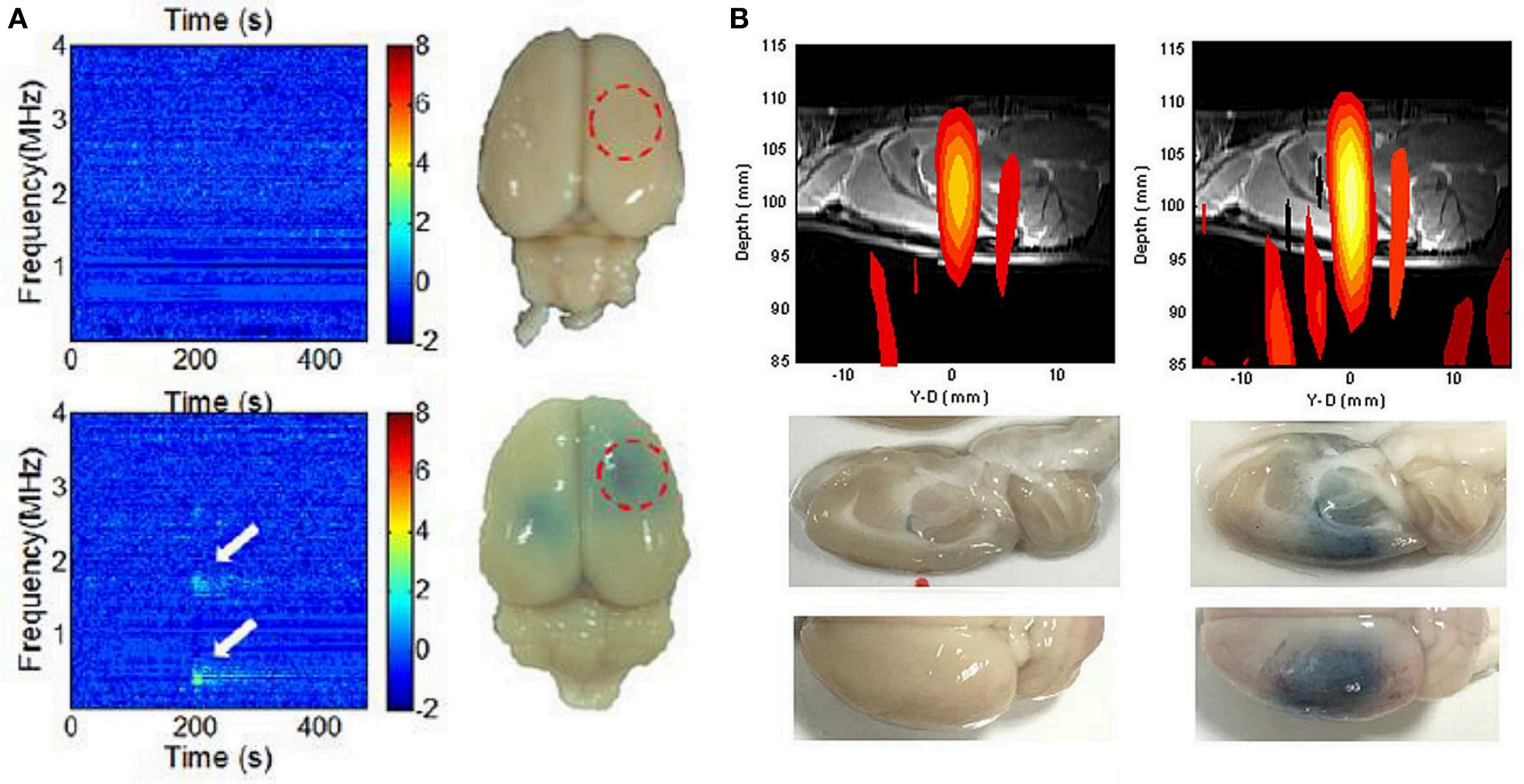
Intraoperative monitoring and guidance of focused ultrasound-induced BBB opening. (A) Detection of subharmonic/ ultraharmonic of backscattered spectrum for real-time BBB opening monitoring (Tsai et al., 2016). (B) Dual transmit/receive mode ultrasound phase array to intraoperatively reconstruct focal beam for ultrasound energy guidance (Liu et al., 2018).
